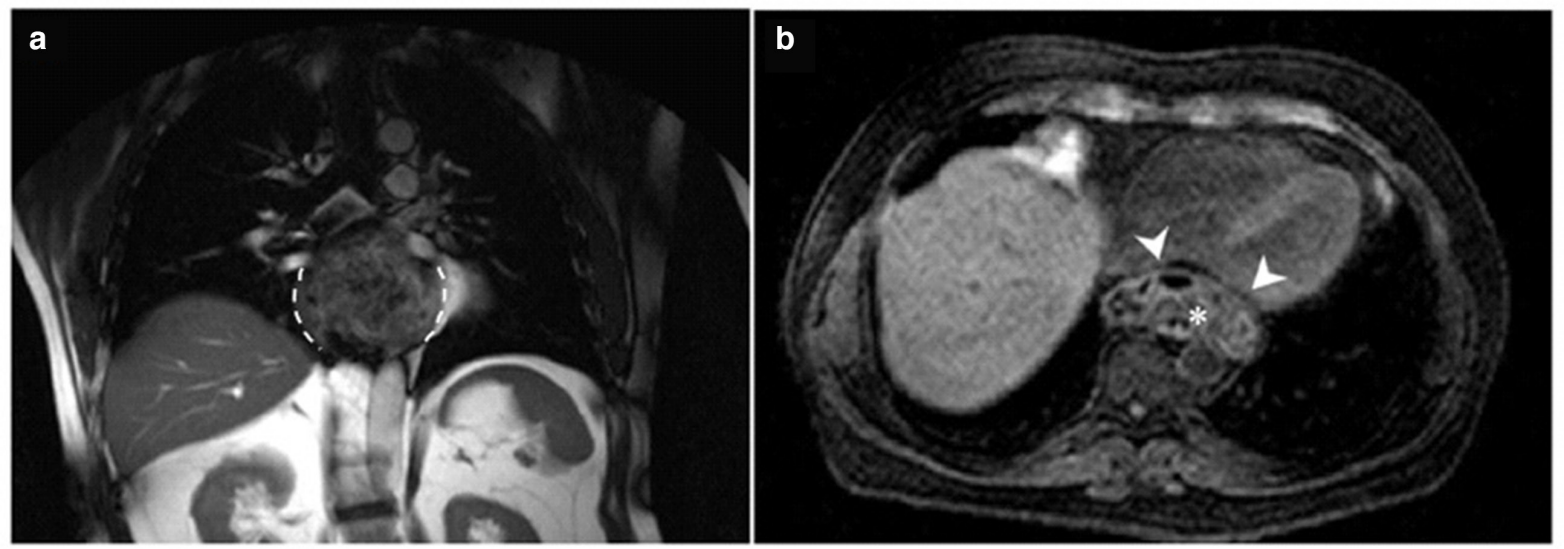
(A) Coronal *T*_1_W MRI demonstrated the left-sided large epiphrenic diverticulum of the esophagus (dashed lines); (B) axial thrive MRI showed the esophageal diverticulum compressing the left atrium and ventricle (arrowhead); it also evidenced the uneven content of the diverticulum, presumably food debris (asterisk).
